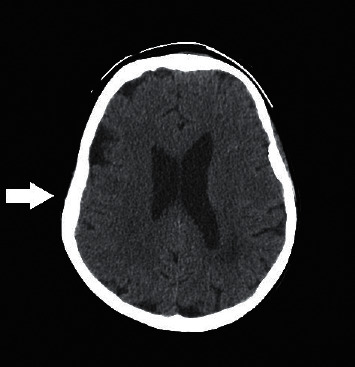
Cranial CT showing marked cerebral and cerebellar atrophy.
